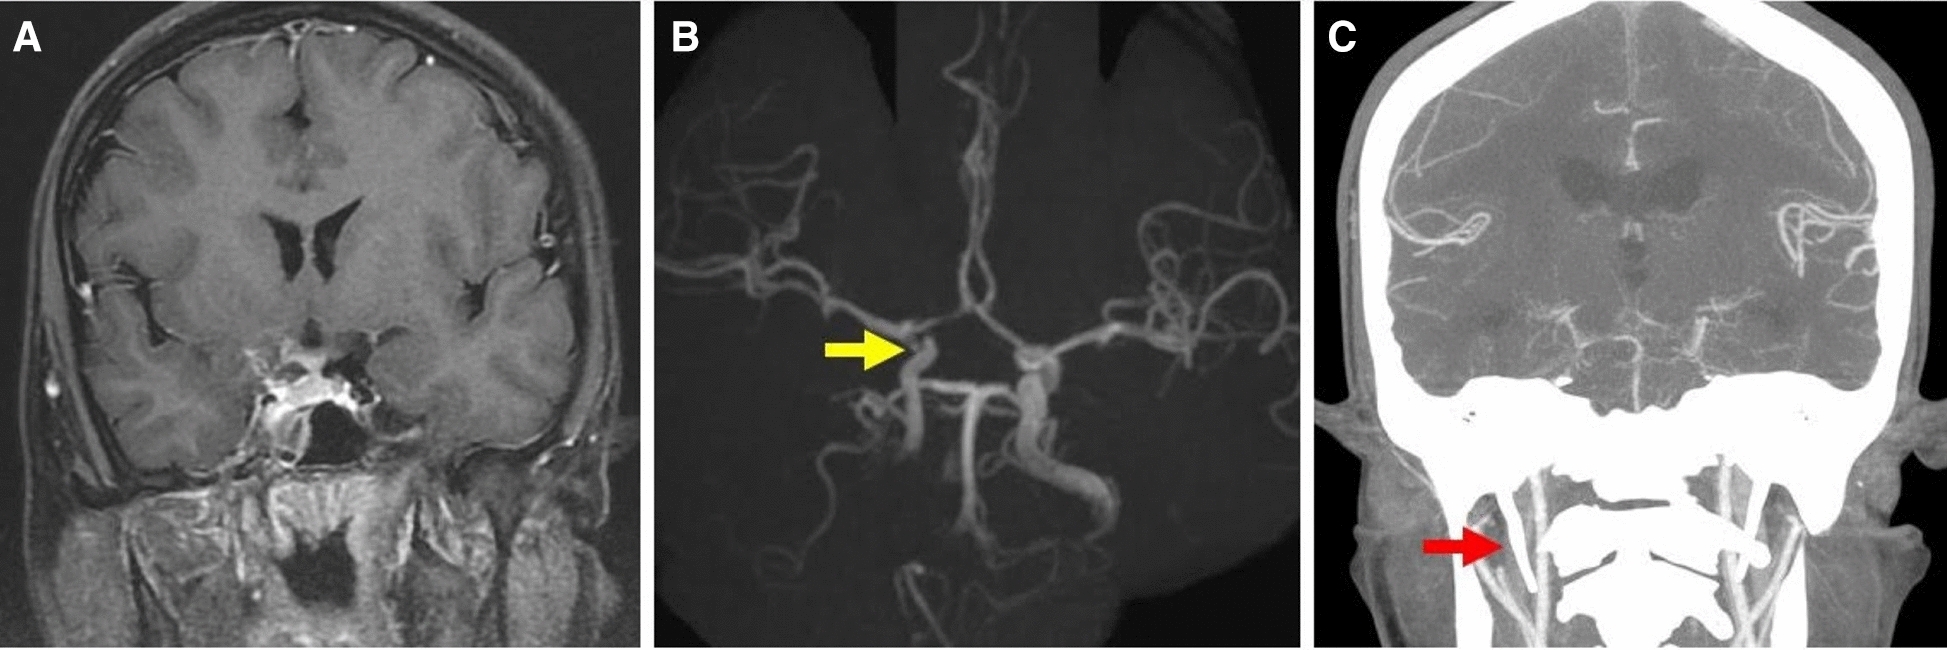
A Brain magnetic resonance imaging showing acute sphenoid sinusitis with right side cavernous sinus thrombosis and internal carotid artery stenosis. B Pre-operative magnetic resonance angiography demonstrating right internal carotid artery stenosis (*arrow*). C Post-operative computed tomography angiography showing reduced flow and a thrombus in the right internal jugular vein (*arrow*)
